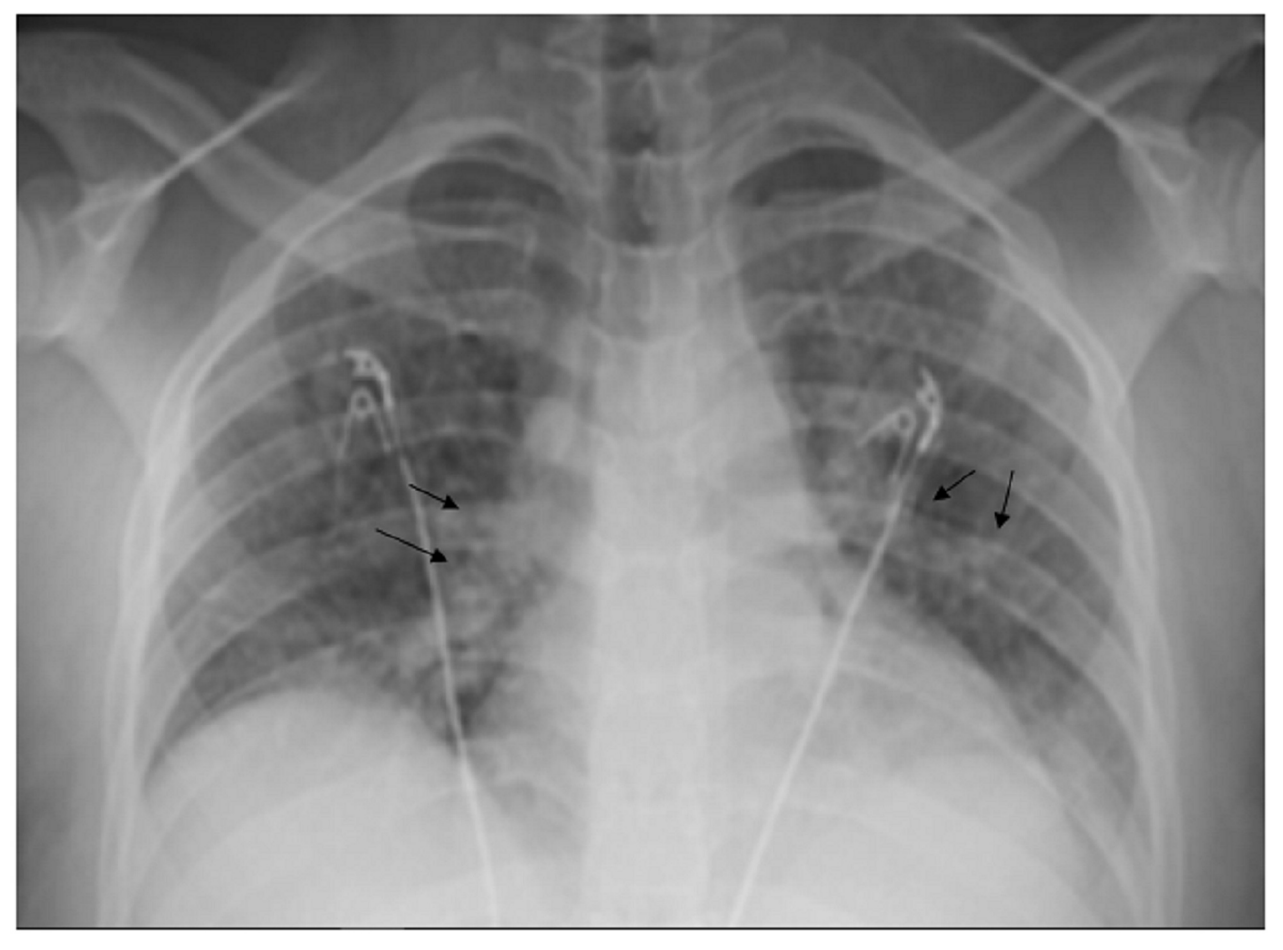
Chest X-ray showing bilateral interstitial and airspace opacities
